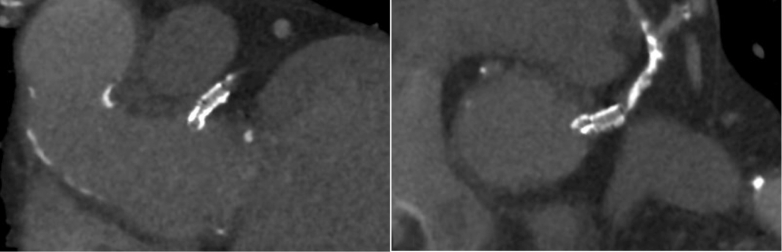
Postprocedural Computed Tomography Showing Orthogonal Views of Stent
